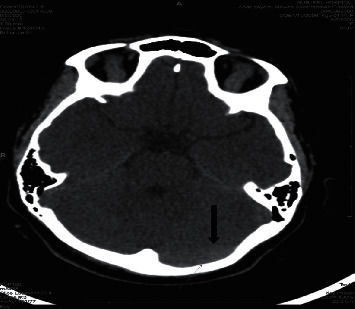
Postcontrast CT axial cut shows filling defect in the transverse sinus suggesting thrombosis (arrow).
